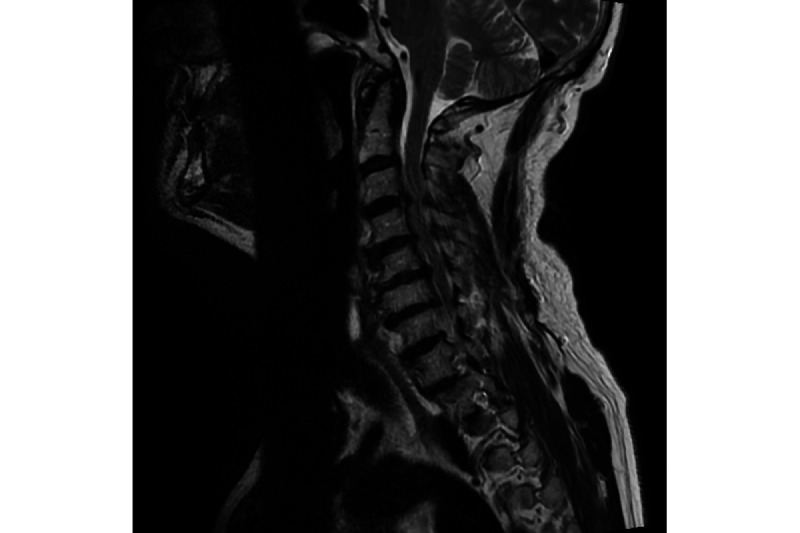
T2-weighted sagittal magnetic resonance imaging showing significant radiological worsening of cervical cord compression at C3-C7 levels despite unchanged symptoms and modified Japanese Orthopaedic Association clinical severity score.
